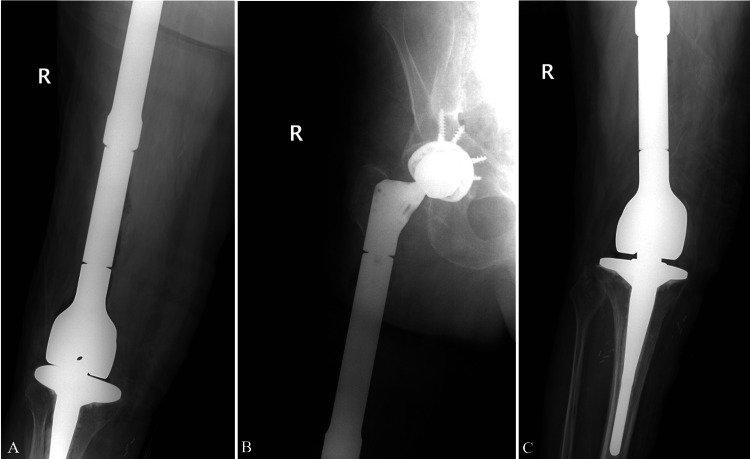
Immediate post-operative anterior-posterior (AP) x-rays of the femur (A), hip (B), and knee (C).
